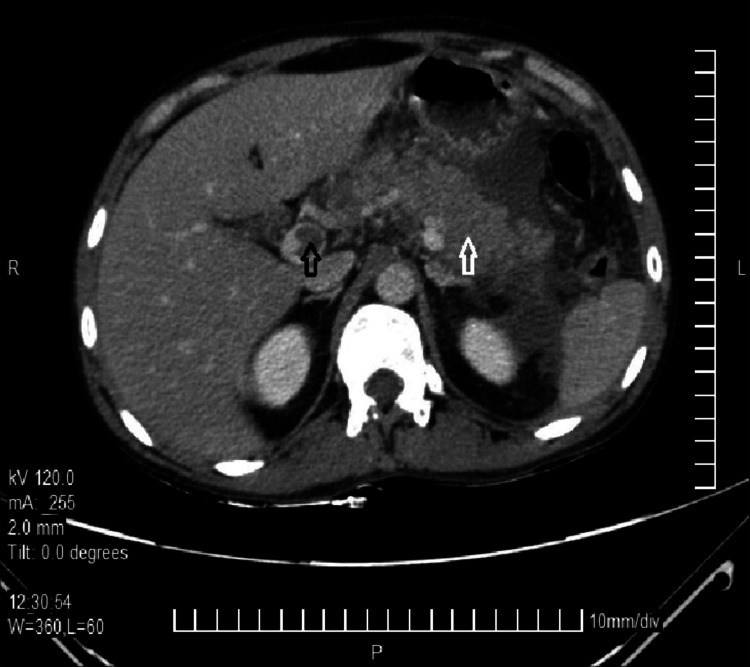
Contrast-enhanced CT scan showing EHPVO Black arrow shows thrombosis of the portal vein and the white arrow shows cavernous transformation of the portal vein and congestion
